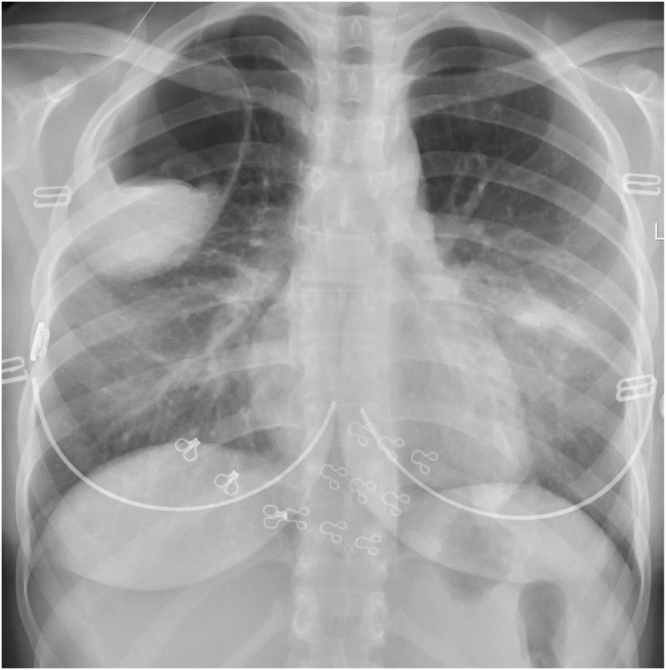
Chest radiograph showing a well-defined cystic lesion in the upper lobe of the right lung.
